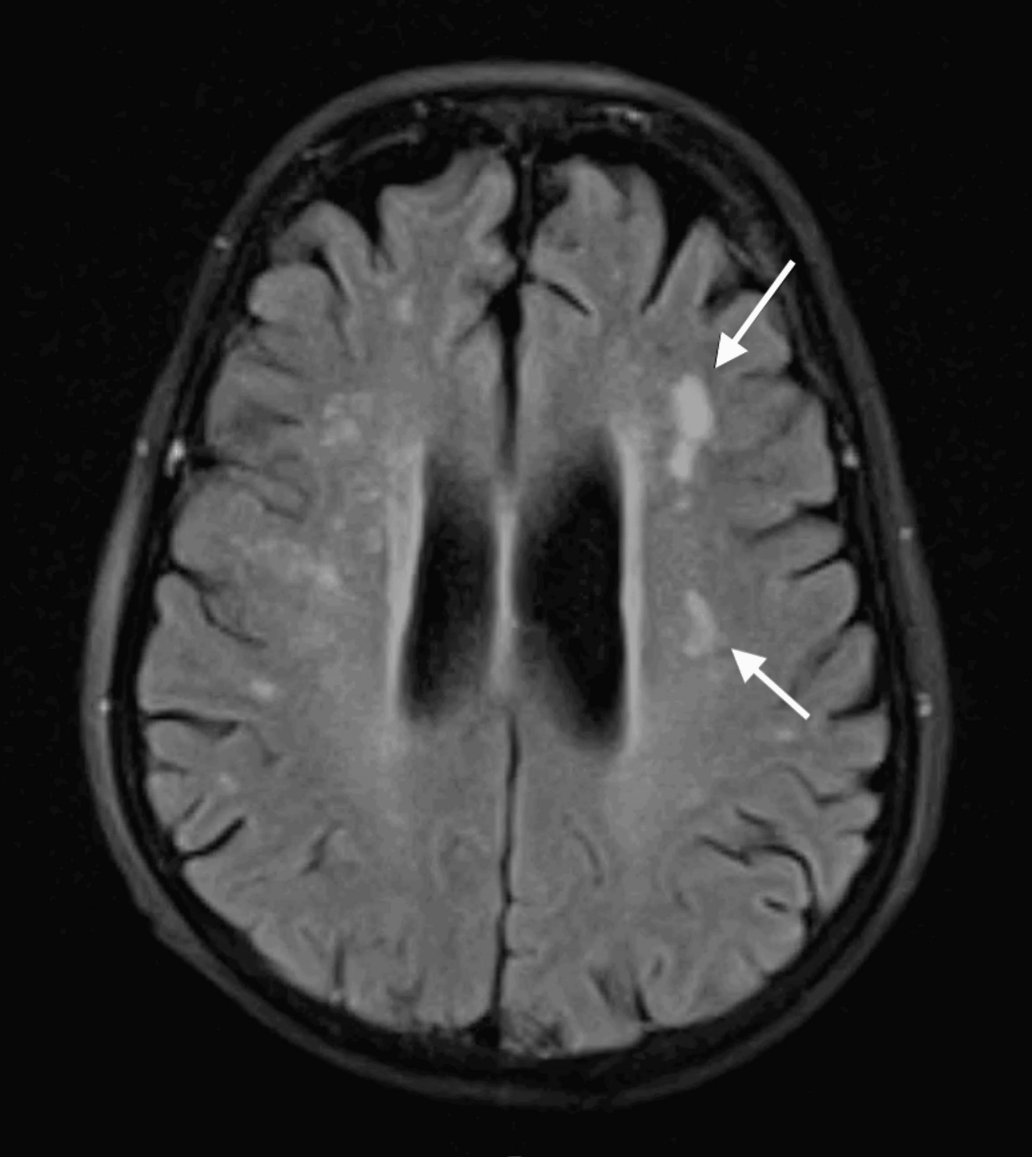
Brain MRI showing asymmetric, non-confluent supratentorial white matter hyperintensities without diffusion restriction, predominantly involving the periventricular regions MRI: magnetic resonance imaging
